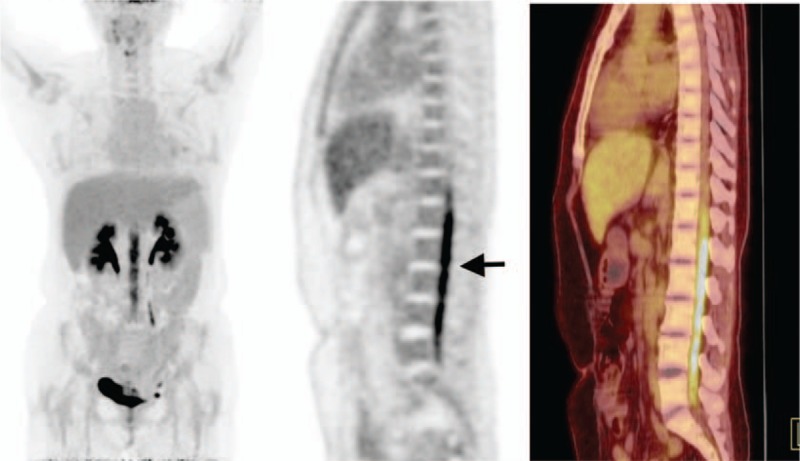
PET/CT images. After 3 cycles of R-CHOP chemotherapy, PET/CT scan showed hypermetabolic lesion in T12-L5 vertebral levels of the spinal cord (*arrow*).
